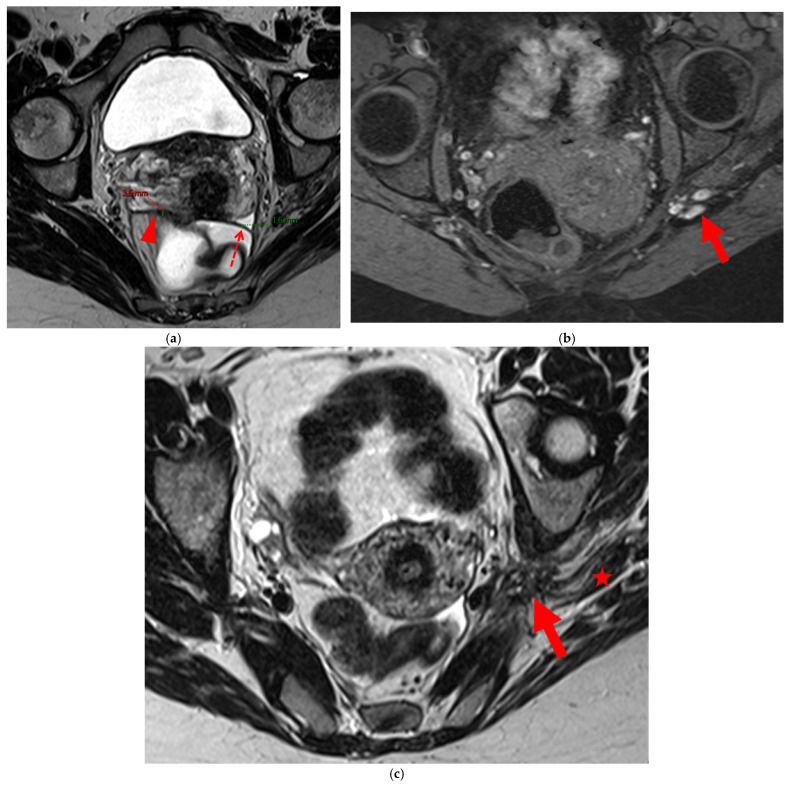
Pelvic MRI scan of a patient with a HTD type 6 USL. (a) Axial T2WI shows a visible but thin (1.6 mm) left USL (*dashed arrow*) and a thick (3.5 mm) but smooth right USL (*arrowhead*), initially classified as a type 2 left USL and a type 3A right USL. (b) Axial fat-suppressed T1WI reveals hyperintense hemorrhagic spots within the left sciatic nerve (*arrow*). (c) Axial T2WI demonstrates spiculated nodularity within the left sciatic nerve (*arrow*), leading to reclassification as type 6 left USL due to this so-called “visceral” nerve involvement. Note the neurogenic amyotrophy (*red star*) of the left piriformis and gluteal muscles, including the gluteus maximus, gluteus medius, and gluteus minimus.
